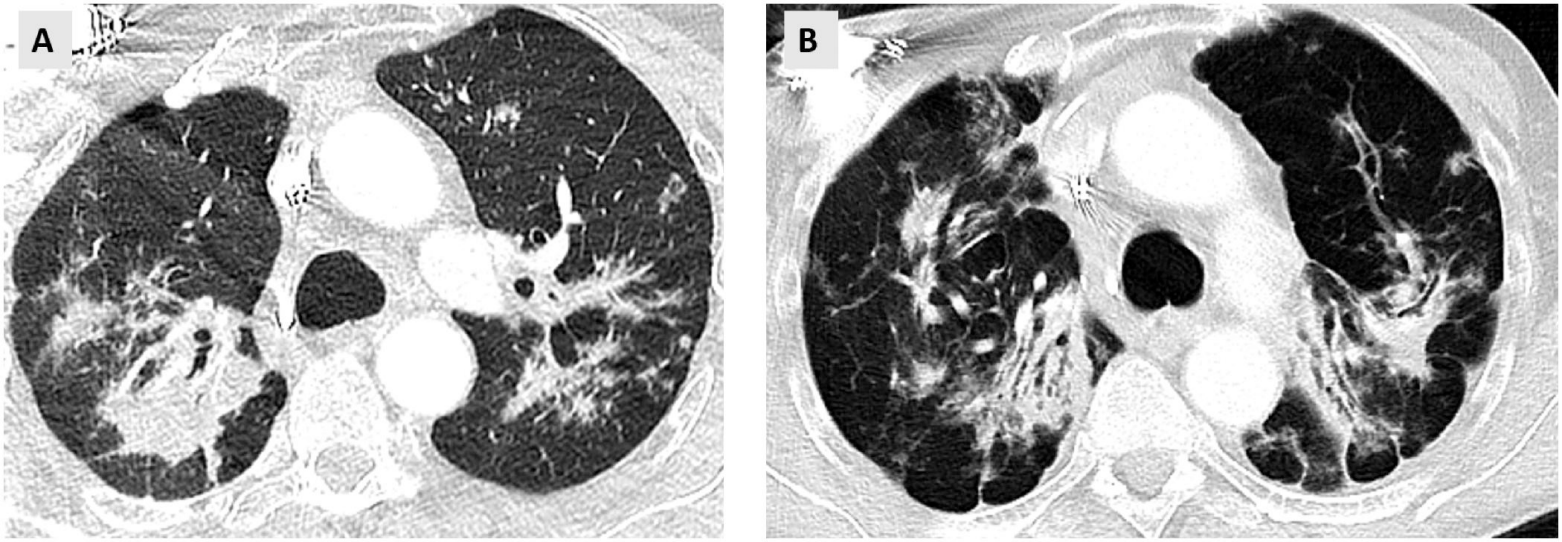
Axial CT scan sections through the upper lobes of a patient with pulmonary sarcoidosis. (A) CT image from 2016 showing a typical pattern with perilymphatic nodules in the left upper lobe and a predominant bronchocentric consolidation in the right upper lobe. (B) Follow-up CT a few years later shows signs of progressive fibrosis with progressive volume loss and posterior distortion of the upper lobe bronchi.
